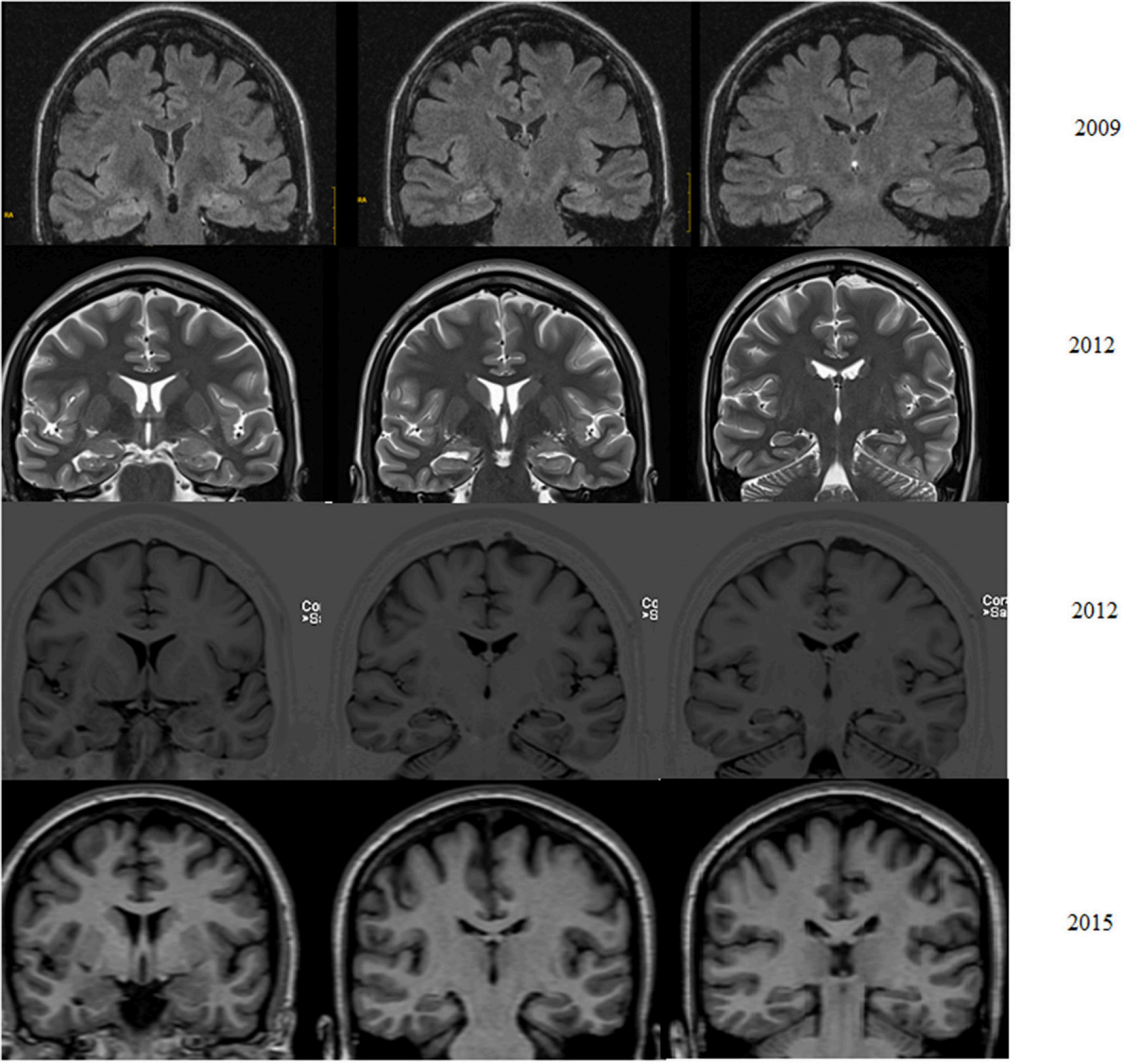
Patient with pharmacoresistant temporal lobe epilepsy from age 25. Five years later suffers weekly focal impaired awareness seizures but no memory decline. Left hippocampal tail atrophy is found on 2012 MRI and confirmed in 2015 (Coronal T1).
